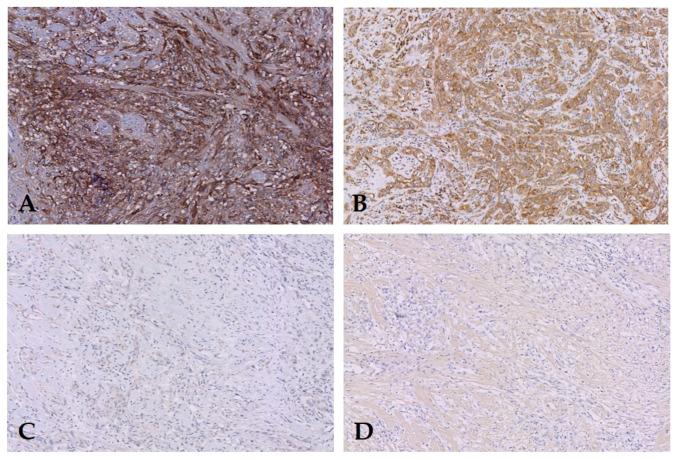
Primary tumor of one patient with (A) EGFR and (B) VEGF expression but absence of (C) prostate-specific membrane antigen (PSMA) and (D) EpCAM expression. Original magnification 200×.
